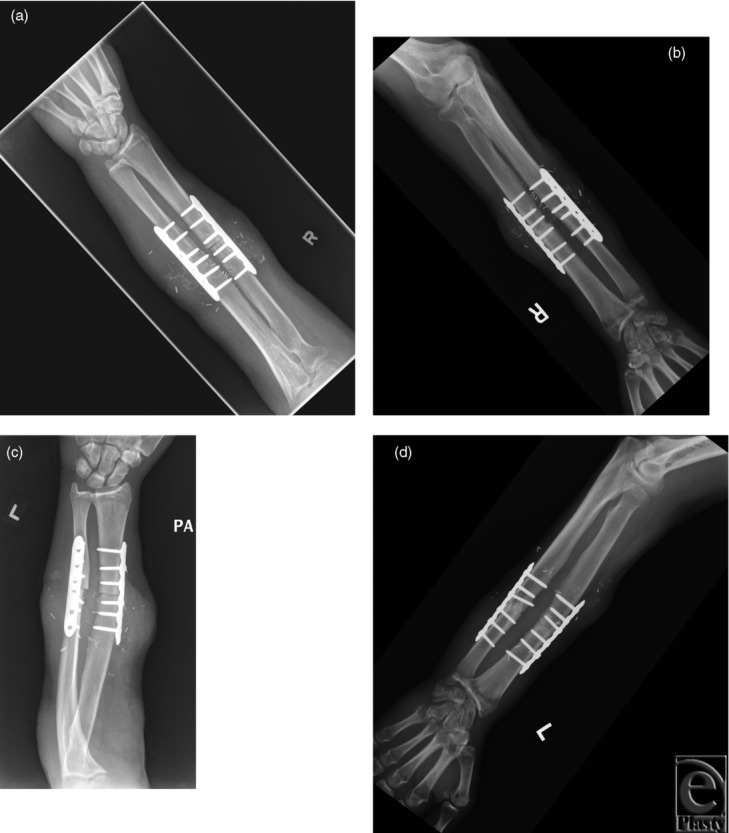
Bilateral elbow radiographs. Frontal radiographs of the bilateral forearm of subject 2 at 1 (*a*, *c*) and 9 months (*b*, *d*) following transplantation demonstrates maturing callus formation and osseous bridging of the radius and ulna at the transplant site. There is interval improvement in soft tissue swelling. No hardware complication is present. Alignment at the elbow and wrist is anatomic.
